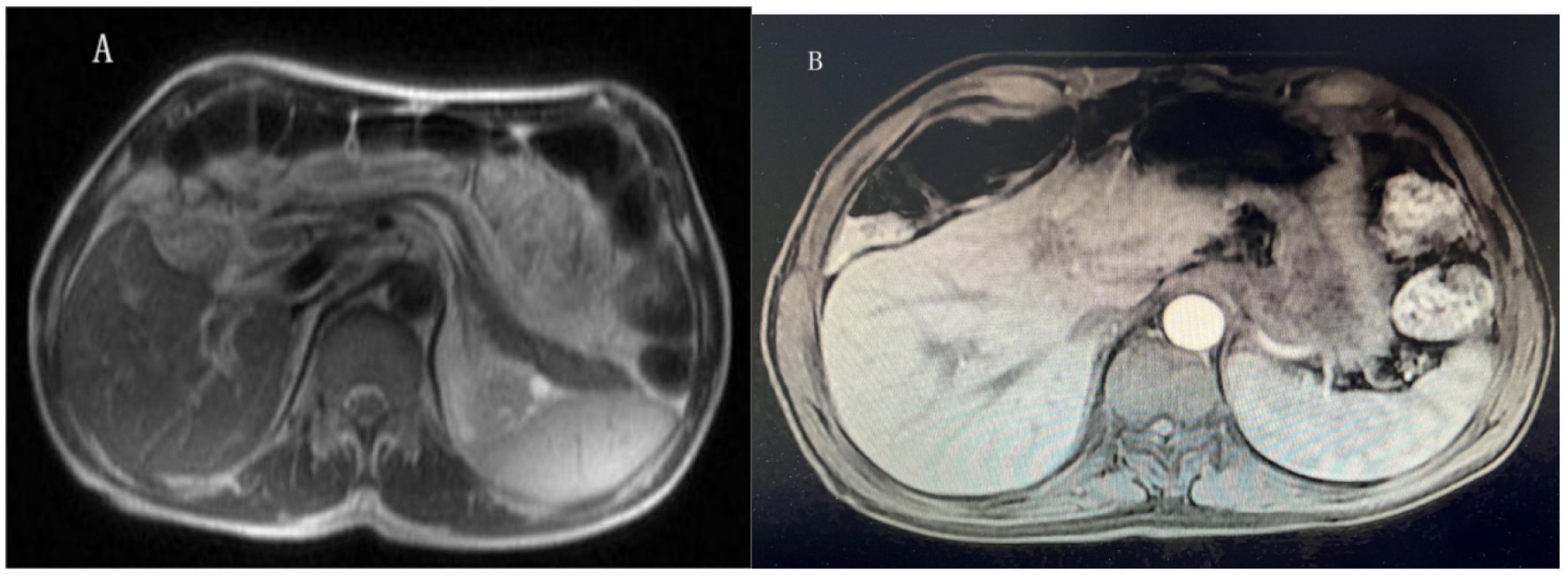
Liver magnetic resonance imaging (MRI). (A, B) Showed the hepatic hemangioma, multiple hepatic cysts, perisplenic effusion, edema of the Glisson sheath and effusion in the gallbladder fossa.
